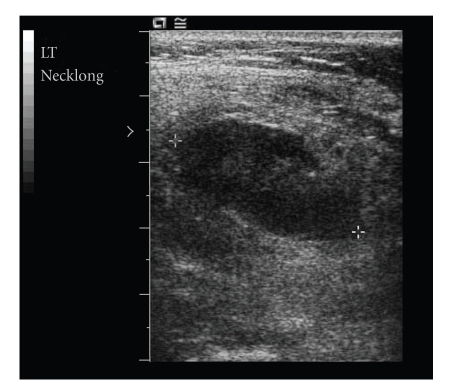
Ultrasonogram of the largest lymph node at the site of cervical swelling. The lymph node was a solid mass measuring 2.6 × 3.1 cm.
